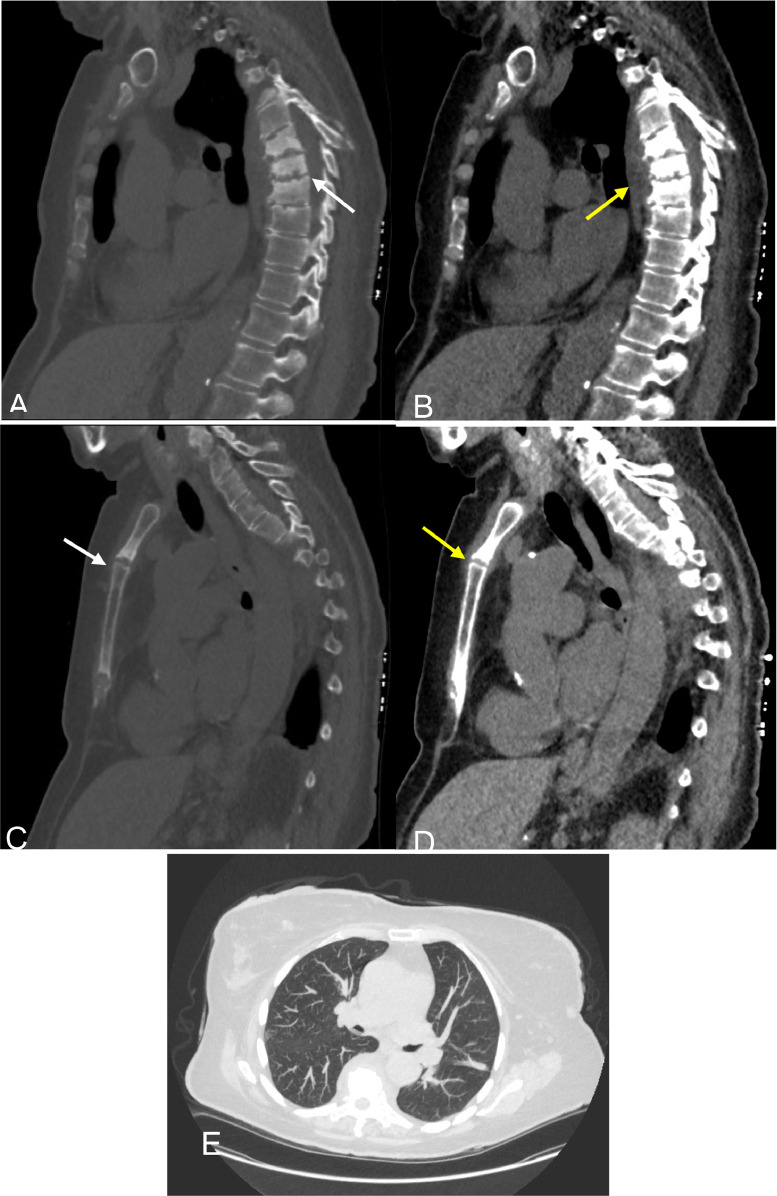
CT Chest: (A) new multilevel sclerotic vertebral lesions noted at T5-T8 with endplate irregularity (white arrow) (B) and associated paravertebral soft tissue phlegmon (yellow arrow). (C) Sclerosis (white arrow) and (D) soft tissue thickening (yellow arrow) also seen at the manubriosternal junction. (E) No pulmonary nodules, consolidation, or adenopathy demonstrated.
